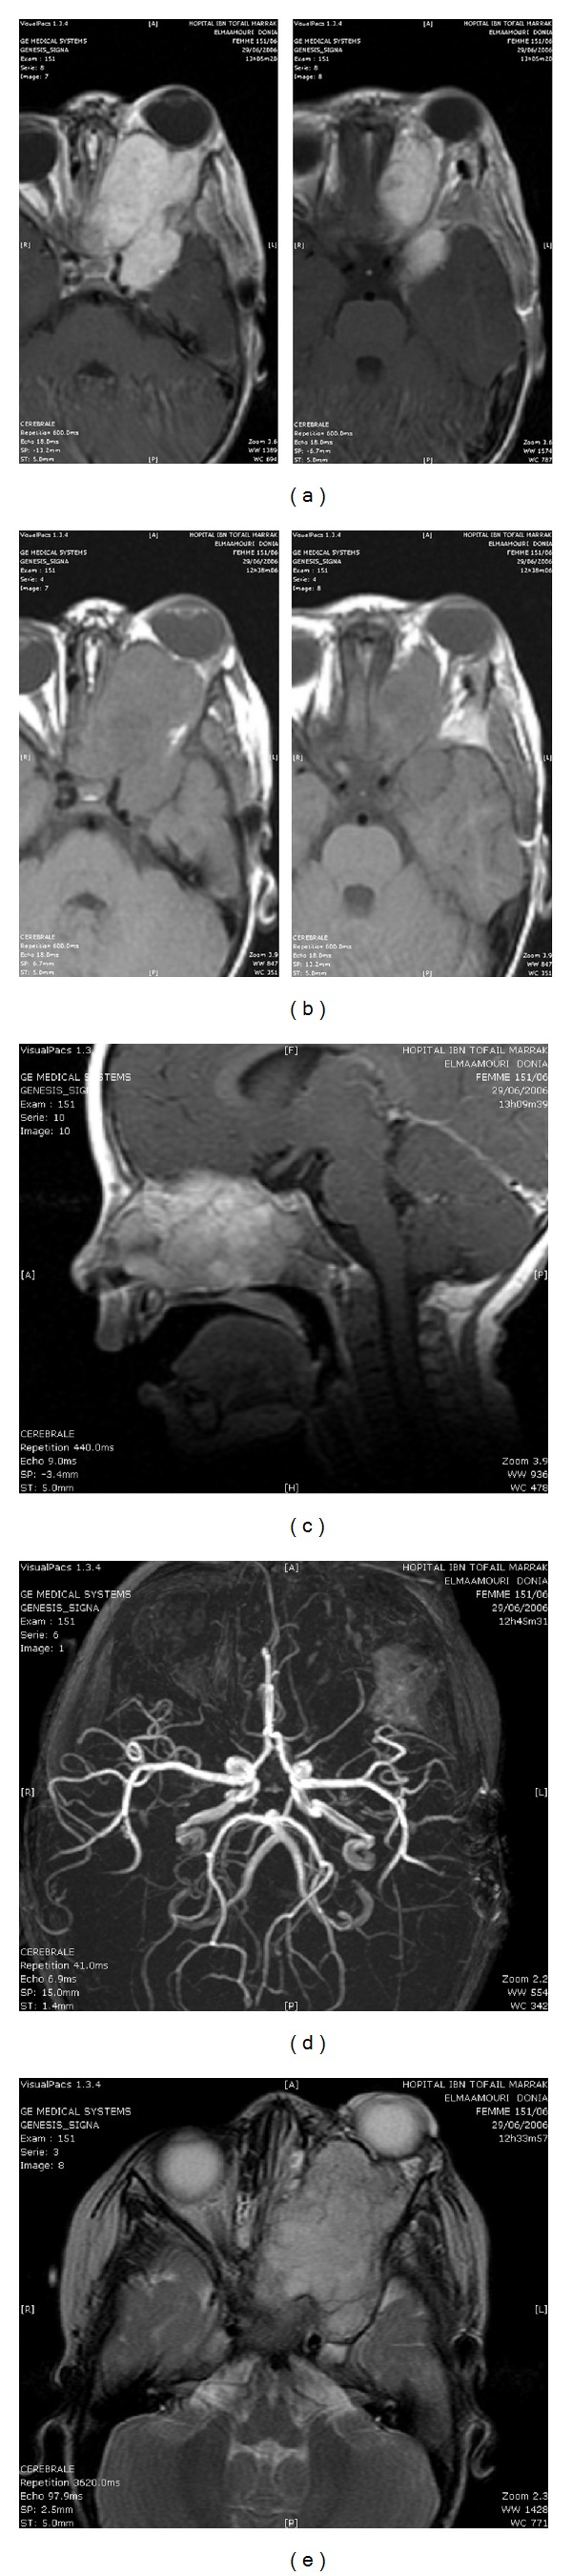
Magnetic resonance imaging in the axial (a, b, and e) and sagittal (c) planes showing the tumoral process in low signal in T1 (b) and high signal in T2 weighted images (e) as well as intense and homogeneous enhancement after contrast administration (a, c). The angio RMI sequence (d) shows the vascular character of the tumor (d).
